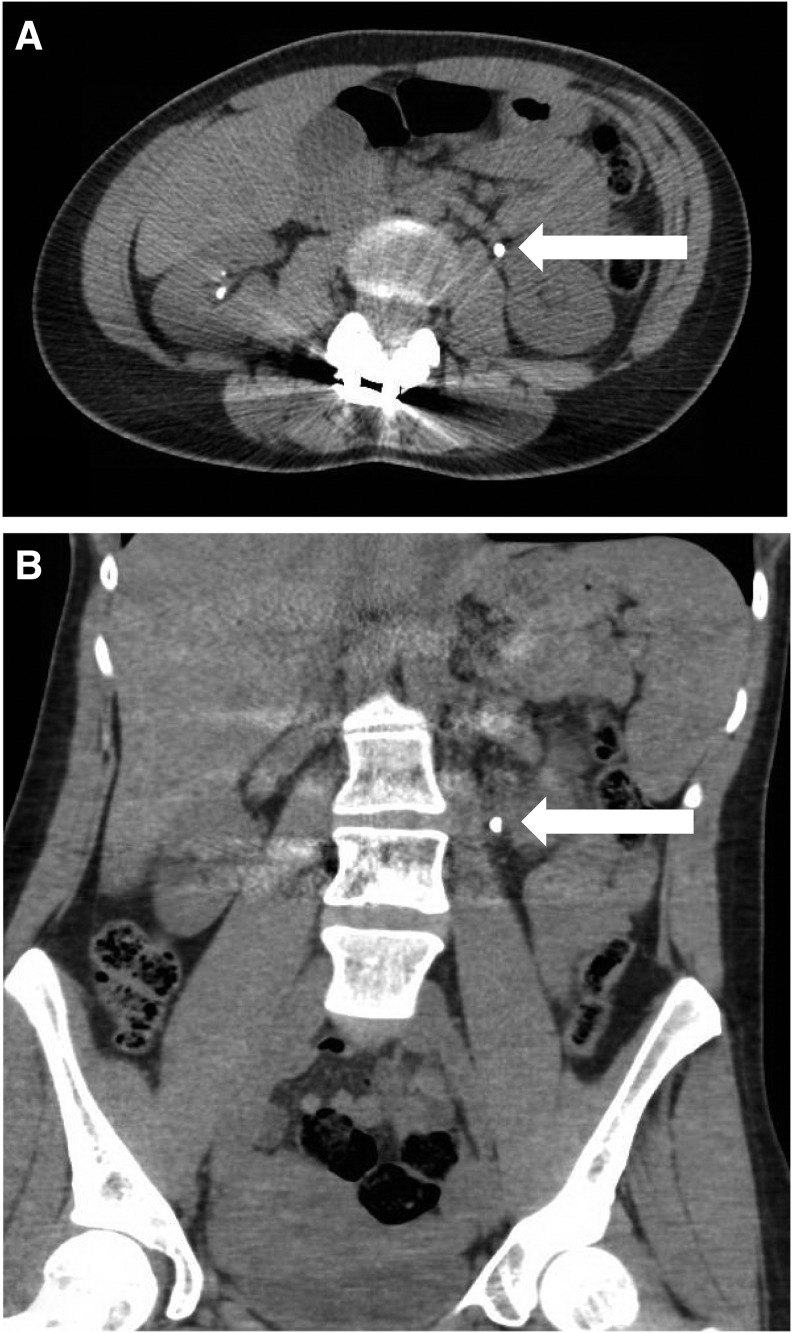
Representative selection of CT abdomen and pelvis images showing location of obstructing proximal ureteral stone, including axial (A) and coronal (B) cuts.
